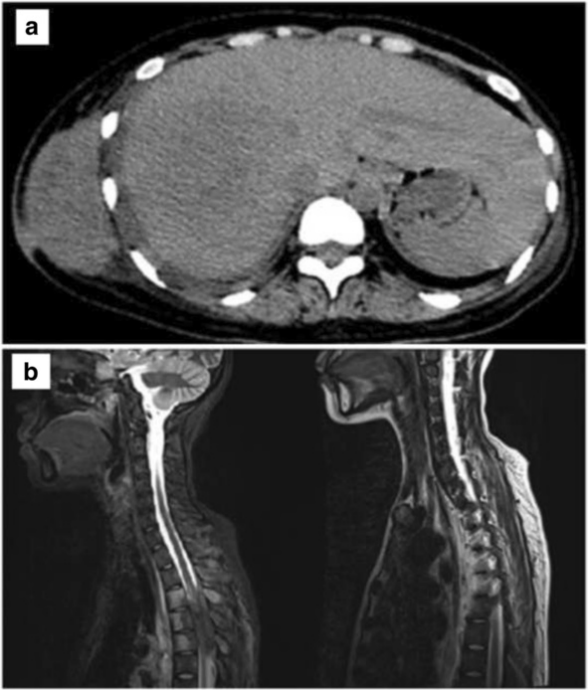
Results of CT and MRI (January 2015) for the chest. The CT shows a soft tissue mass (12.5 cm × 5.9 cm) around a small pneumatosis in the right chest wall, and a reduced density in the right liver lobe near the diaphragmatic top (a). The MRI shows lesions invaded the spinal channel and spinal cord (b)
